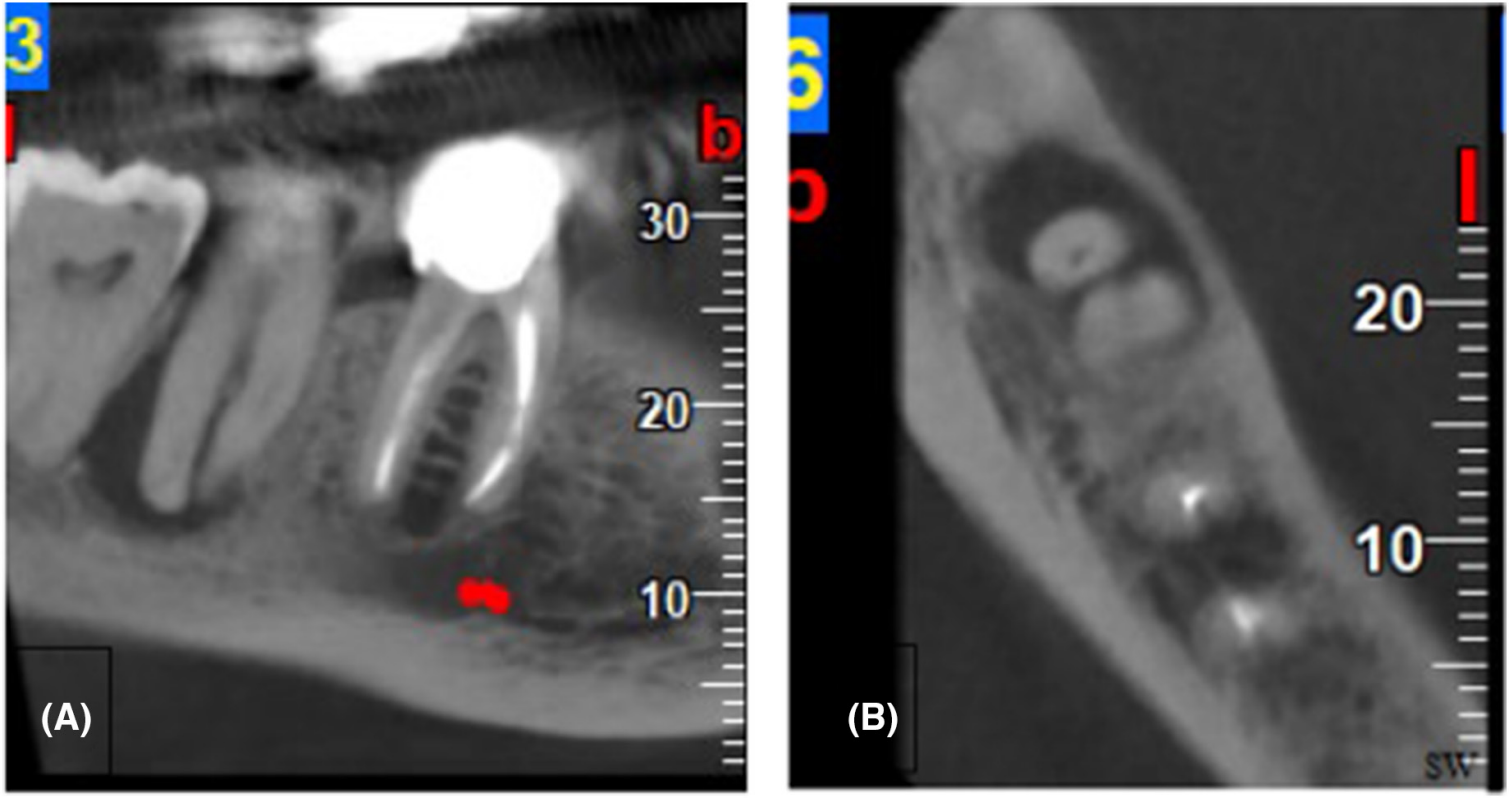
Panoramic‐like reconstructed image (A) and axial view of cone‐beam computed tomography (B) showing a J‐shaped radiolucent lesion.
